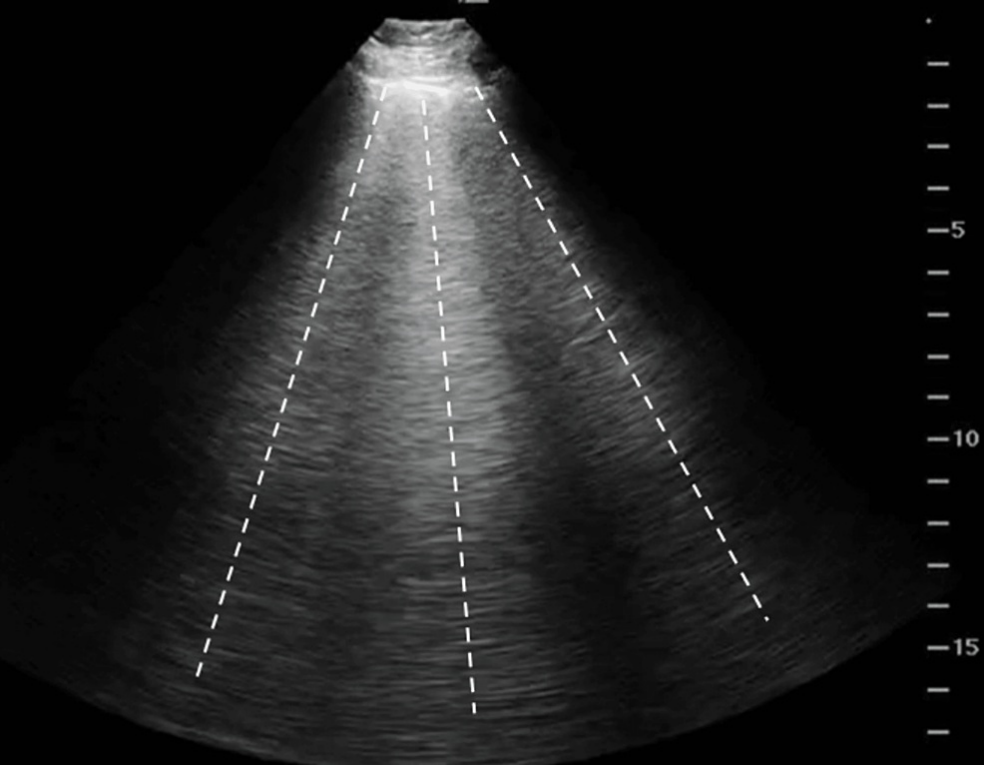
Point-of-care lung ultrasound Point-of-care lung ultrasound demonstrating diffuse b-lines (dotted lines) indicative of pulmonary edema.
